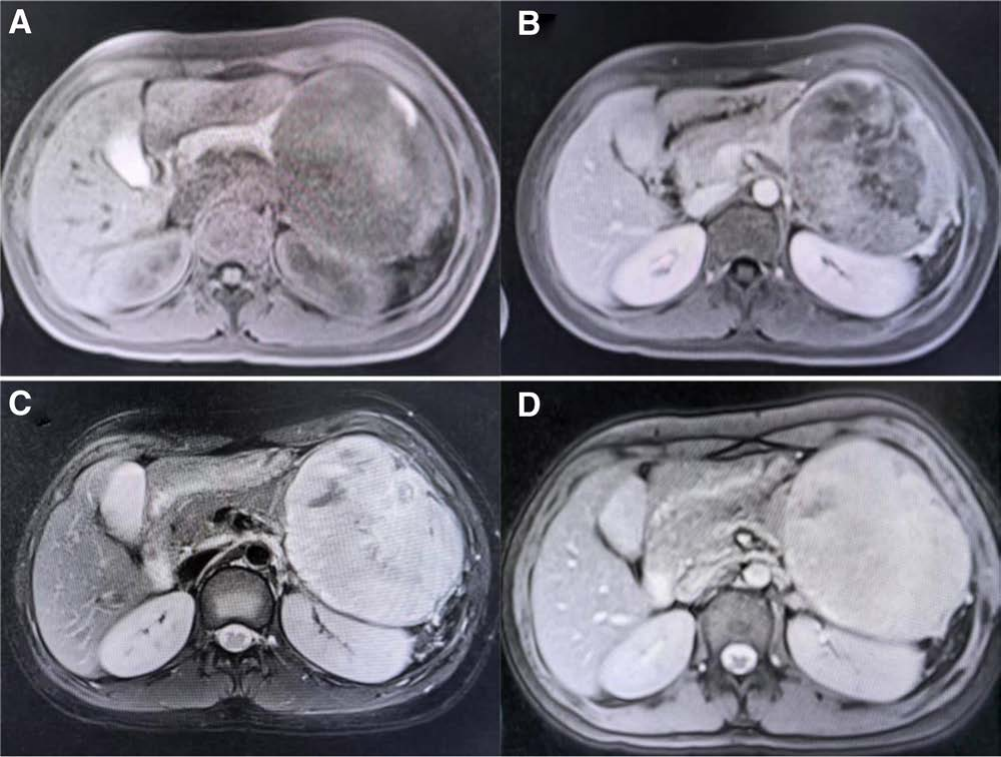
Abdominal MRI revealed large pancreatic mass and altered signal intensity. The mass involved the distal pancreas, pressing on the left kidney and forming a “concave line” on the renal side. (A) T1; (B) T1 + contrast; (C) T2; (D) T2 + contrast. MRI = magnetic resonance imaging.
